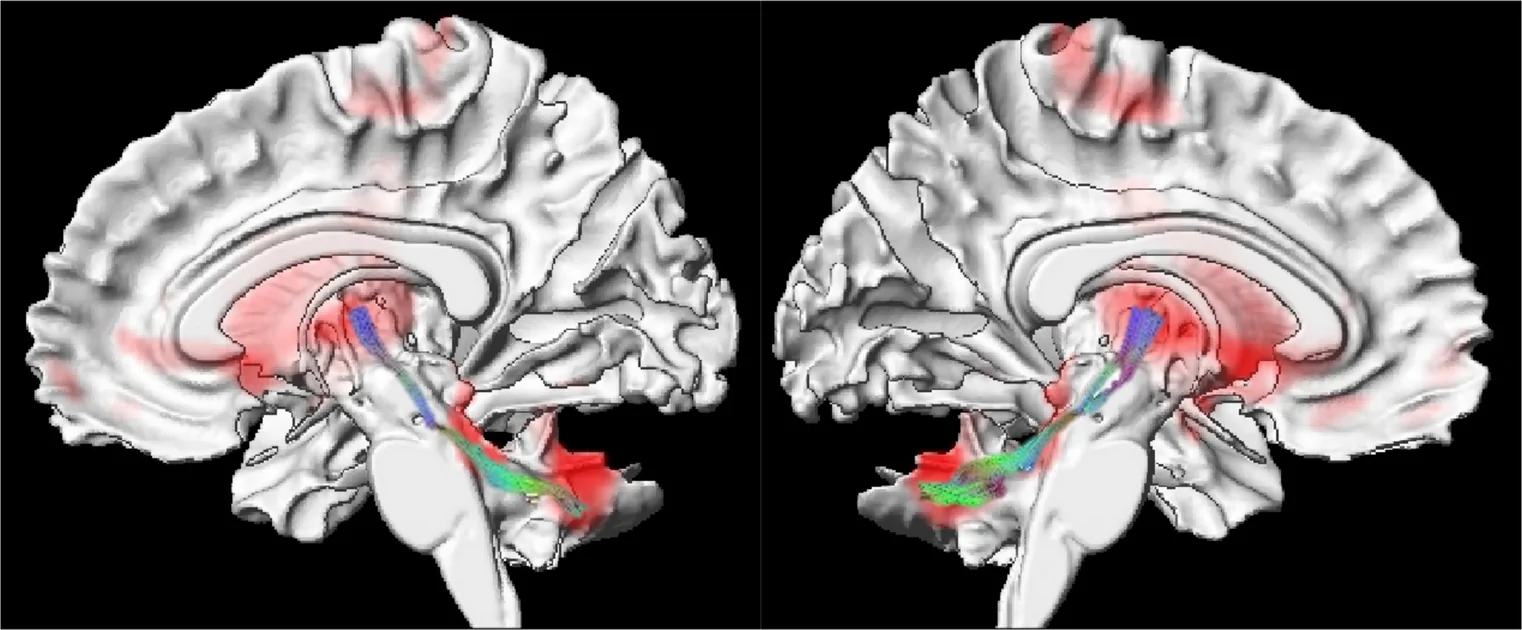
Regional [^18^F]FDG PET hypermetabolism in patients with DLB (JBZ cohort) when compared to healthy controls, sagittal midline view. Dentatorubrothalamic tract (DRTT) was delineated from human connectome data with a seed region placed in the dentate nucleus (Julich-Brain Atlas) and region-of-interests in the contralateral red nucleus (BrainSeg atlas) and thalamus (BrainSeg atlas) [65–67]. Regions of significant differences in hypermetabolism are shown in red, with tractography of the bilateral DRTT overlaid for contextual reference. DLB = Dementia with Lewy bodies; FDG = [^18^F]Fluorodeoxyglucose
